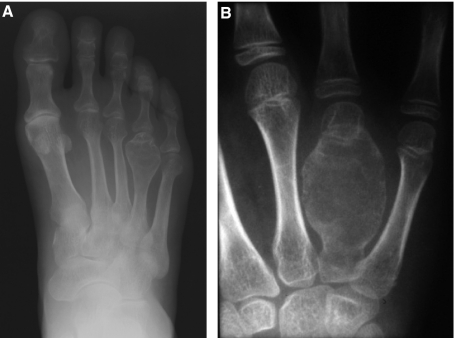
Radiograph of GCT involving the distal fourth metatarsal bone (A) and diaphysis of the fourth metacarpal bone (B). Both lesions are purely lytic with partially sclerotic rim and markedly expanded cortical bone, but with no cortical destruction. The centers of the lesions appear to be centrally located within the bones.
